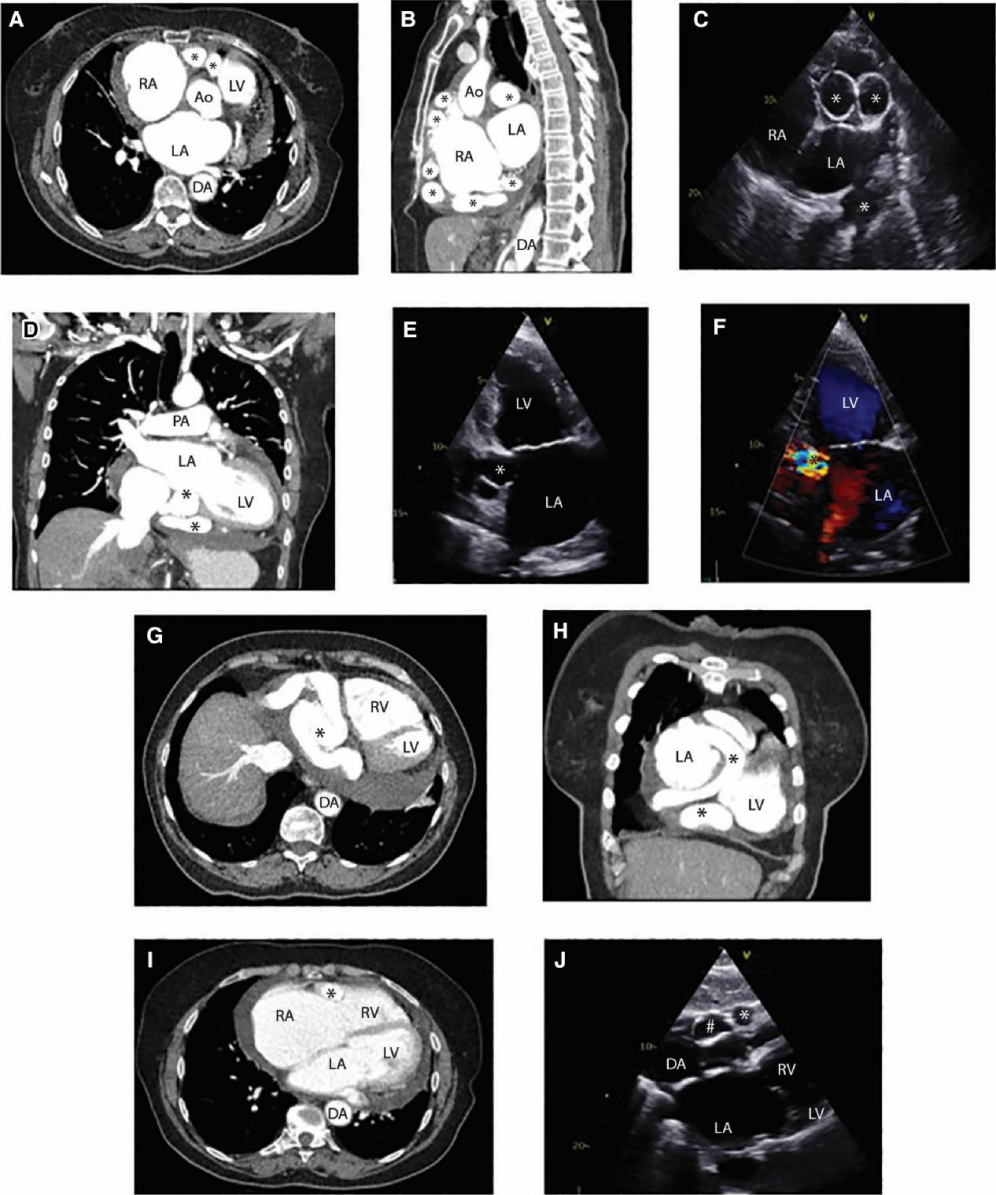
Multiple cross-sectional and transthoracic echocardiographic (TTE) images elucidating the tortuous course of the right coronary artery (RCA) (marked across all images with asterisk). (*A*, *G*, and *I*) Axial oblique slices on CT, (*B*) sagittal oblique view on CT demonstrating cross-sectional slices of the RCA ‘wrapping’ around the heart in relation to the left and right atria, (*C*) high parasternal short axis view on TTE, (*D* and *H*) coronal slices on CT, (*E* and *F*) apical two-chamber view on TTE showing the posterior course of the aneurysmal RCA with turbulent flow seen on colour Doppler. (*J*) Off axis subcostal view on TTE demonstrating the relationship of the RCA anteriorly and termination site, labelled as number sign. RA, right atrium; LA, left atrium; Ao, ascending aorta; LV, left ventricle; RV, right ventricle; DA, descending aorta; PA, pulmonary artery.
